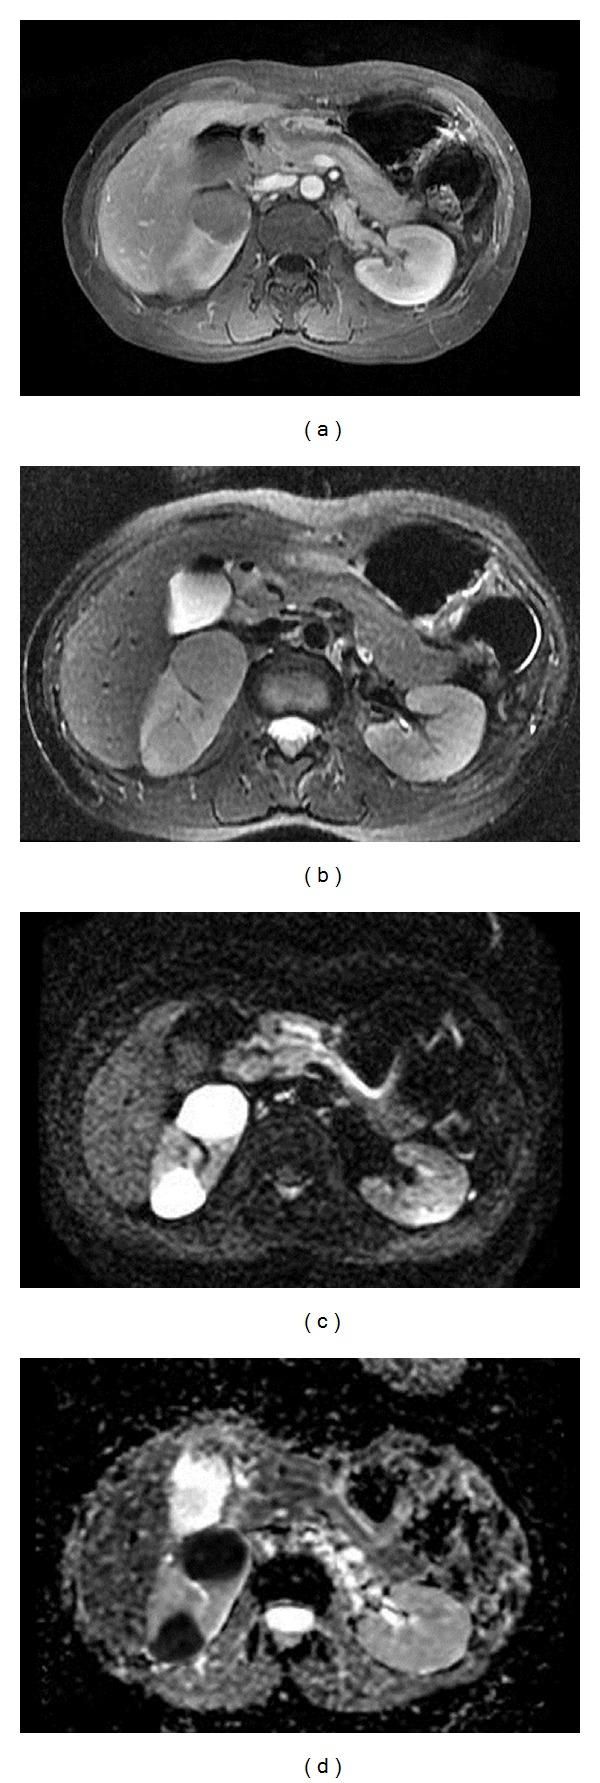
Abdominal magnetic resonance imaging (MRI). Abdominal MRI showed right kidney multiple lesions without enhancement during arterial phase of dynamic study (a), hypointense masses on T2-weighted images (b), and multifocal hypovascular hypercellular solid tumors of the right kidneys with bright lesion in diffusion weighted imaging (DWI) (c) and significantly decreased apparent diffusion coefficient (ADC) (d).
